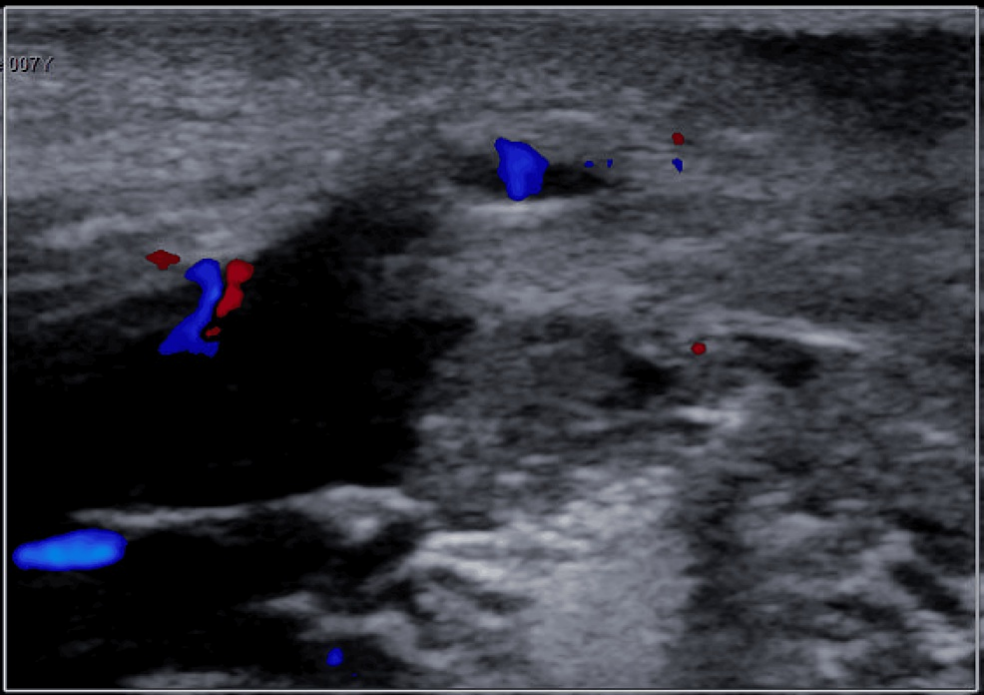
Ultrasound Demonstrating Areas of Thyroglossal Duct Cyst (TGDC) Persistence
